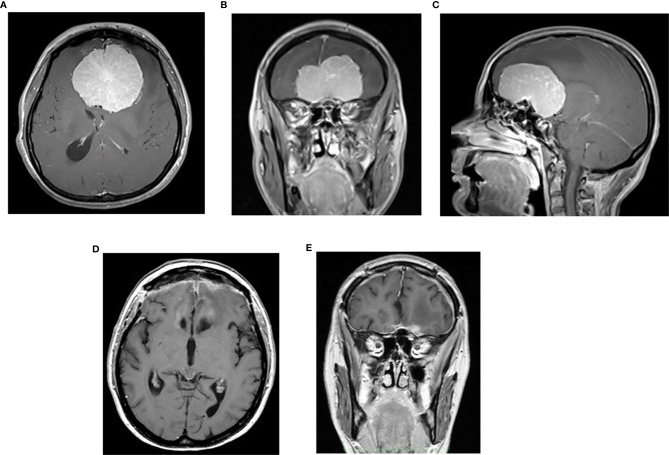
Preoperative post contrast MRI, axial (A), coronal (B) and sagittal (C) sequences of 37-year-old female with large ethmoidal–sphenoidal planum meningioma presenting with visual loss and intracranial hypertension syndrome at 26^th^ week of pregnancy; postoperative post-contrast axial (D) and coronal (E) sequences.
